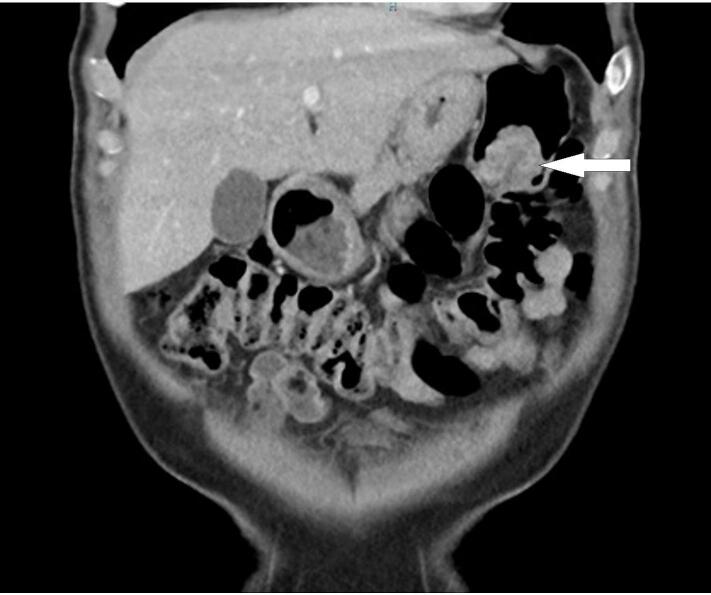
Coronal CT image identifying lesion in the splenic flexure (white arrow).
